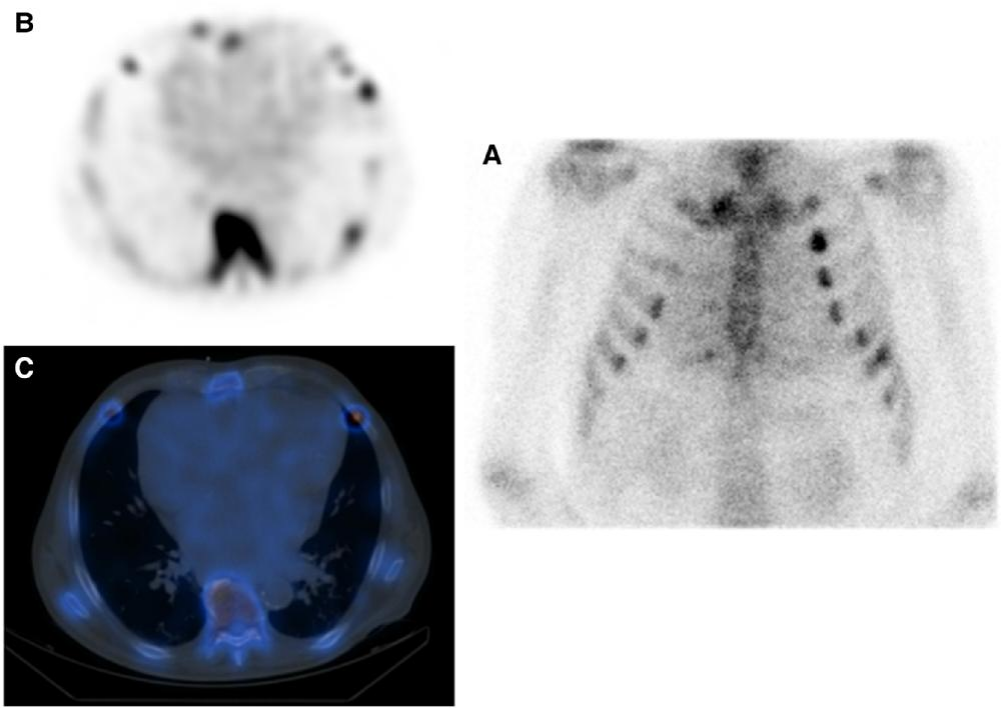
Cardiac scintigraphy and SPECT/CT. Cardiac scintigraphy evidenced grade 1 cardiac uptake consistent with light chain amyloidosis (*A* and *B*) and cardiac single photon emission computed tomography (SPECT/CT) showing cardiac involvement (*C*).
